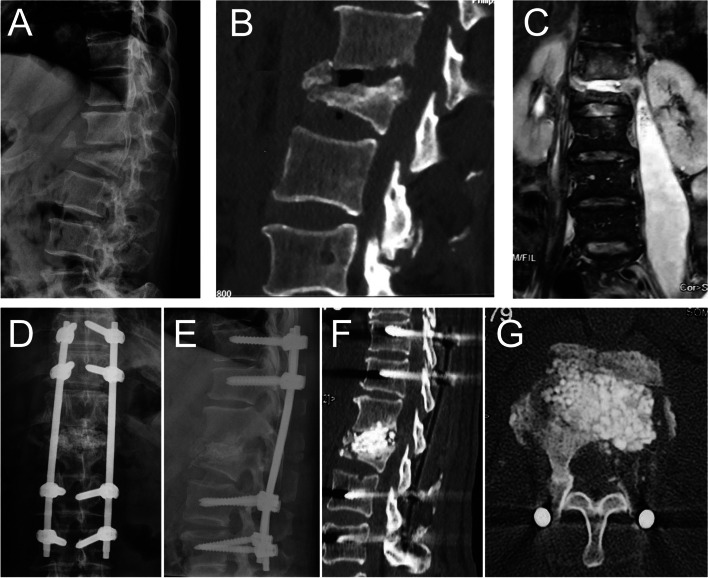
A 66-year-old male with thoracolumbar spondylodiscitis. A,B Preoperative sagittal X-ray and CT showed the collapse of the vertebral body, leading to the kyphosis. C Preoperative coronal MRI showed psoas abscess formation. D,E Postoperative anteroposterior and lateral radiographs after long segmental fixation showed acceptable spinal alignment. F,G Postoperative sagittal and axial CT showed the antibiotic-loaded calcium sulfate beads in the disc space
